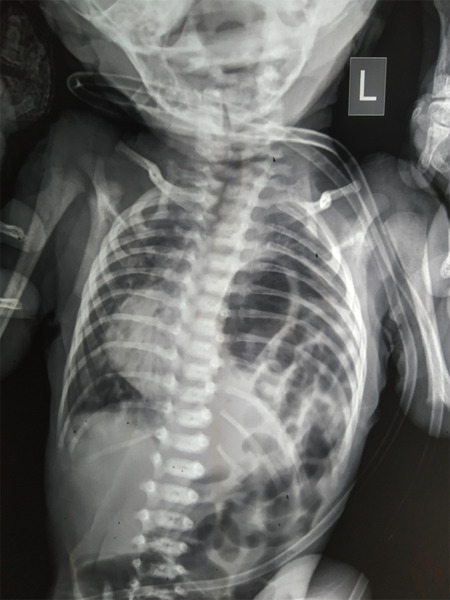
Chest radiograph showing loops of gas filled bowel over the left side of chest suggestive of left sided CDH.
